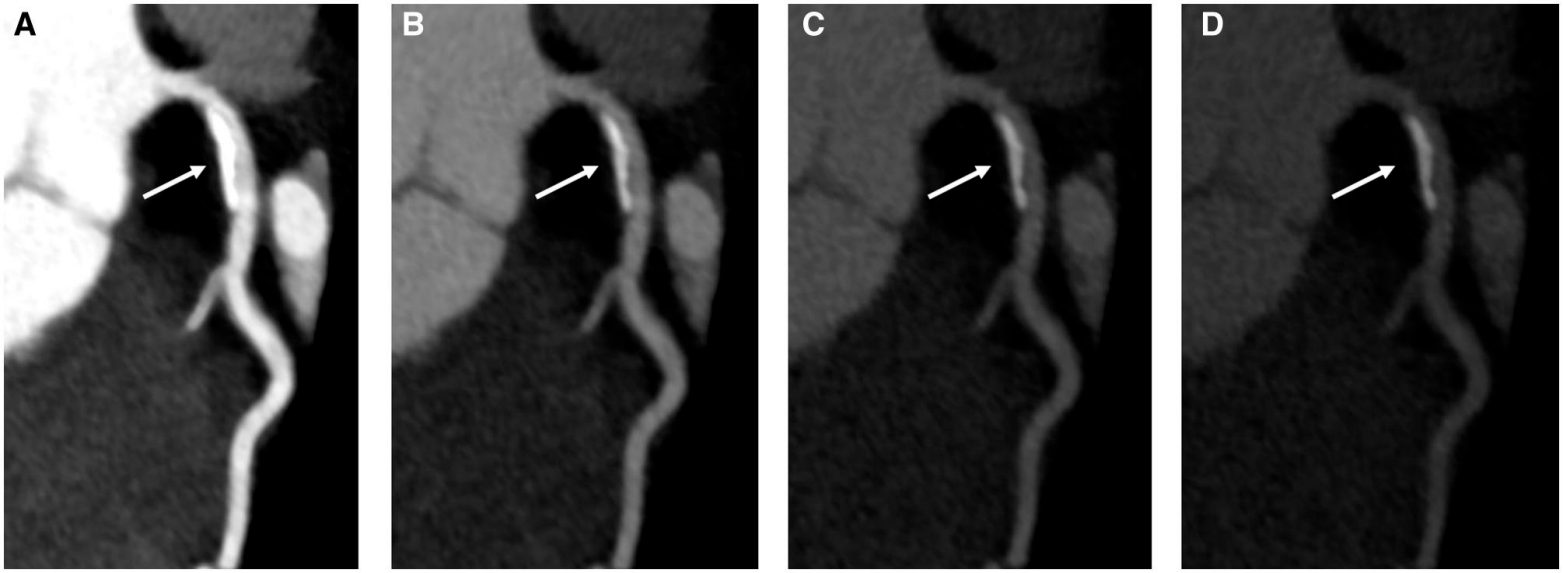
A 56-year-old female patient undergoing multienergy coronary CT angiography with a dual-source photon-counting detector CT. Calcified plaque in the proximal left anterior descending (LAD) artery (arrows) in 40 keV (A), 60 keV (B), 80 keV (C), and 100 keV (D) keV reconstructions. Note the reduction of calcium blooming at higher monoenergetic levels and the reduced iodine contrast.
